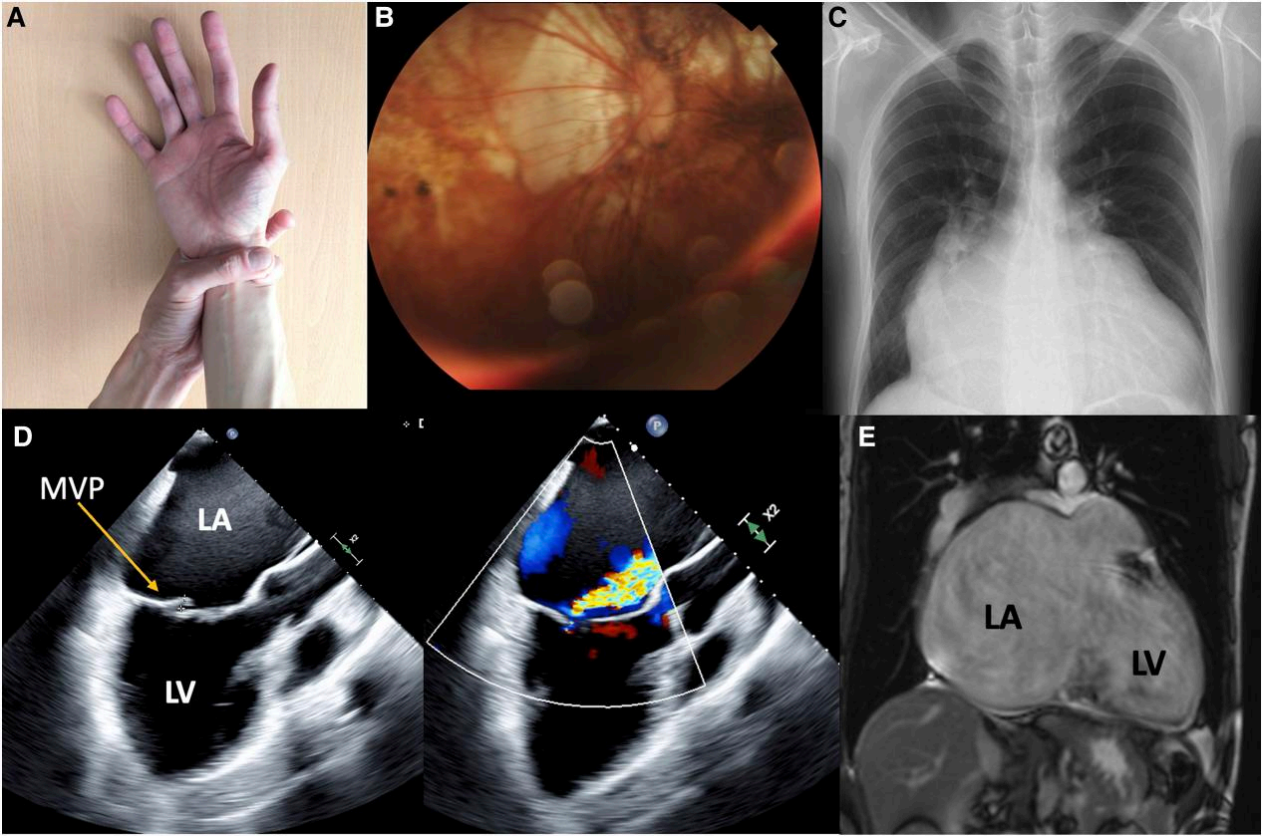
Giant left atrium in a patient with Marfan syndrome. (*A*) Walker–Murdoch wrist sign; (*B*) the funduscopic examination showing myopic choroidosis with chorioretinal atrophy; (*C*) chest X-ray showing cardiomegaly (cardiothoracic ratio 0.81); (*D*) trans-oesophageal echocardiogram (left side) showing giant left atrium comparing left ventricle, with posterior valve prolapse of the mitral valve (arrow), with colour Doppler showing severe regurgitation (right side); (*E*) cardiac magnetic resonance imaging showing giant left atrium and left ventricle in a coronal axis. LA, left atrium; LV, left ventricle; MVP, mitral valve prolapse.
